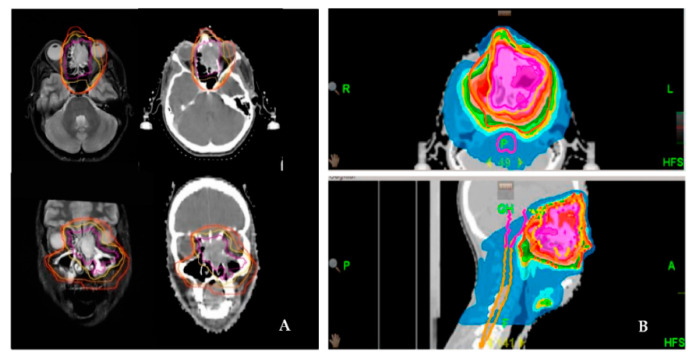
Delineation and dose distribution by helical tomotherapy of a left-sided paranasal carcinoma with orbital involvement. Definitive radiation treatment was performed using a SIB concept on Hi-Art tomotherapy (A). Delineation of the target volume on both CT and MRI scans (magenta: GTV; yellow: SIB-1; orange: SIB-2; red: PTV). (B). Dose distribution as a color wash.
